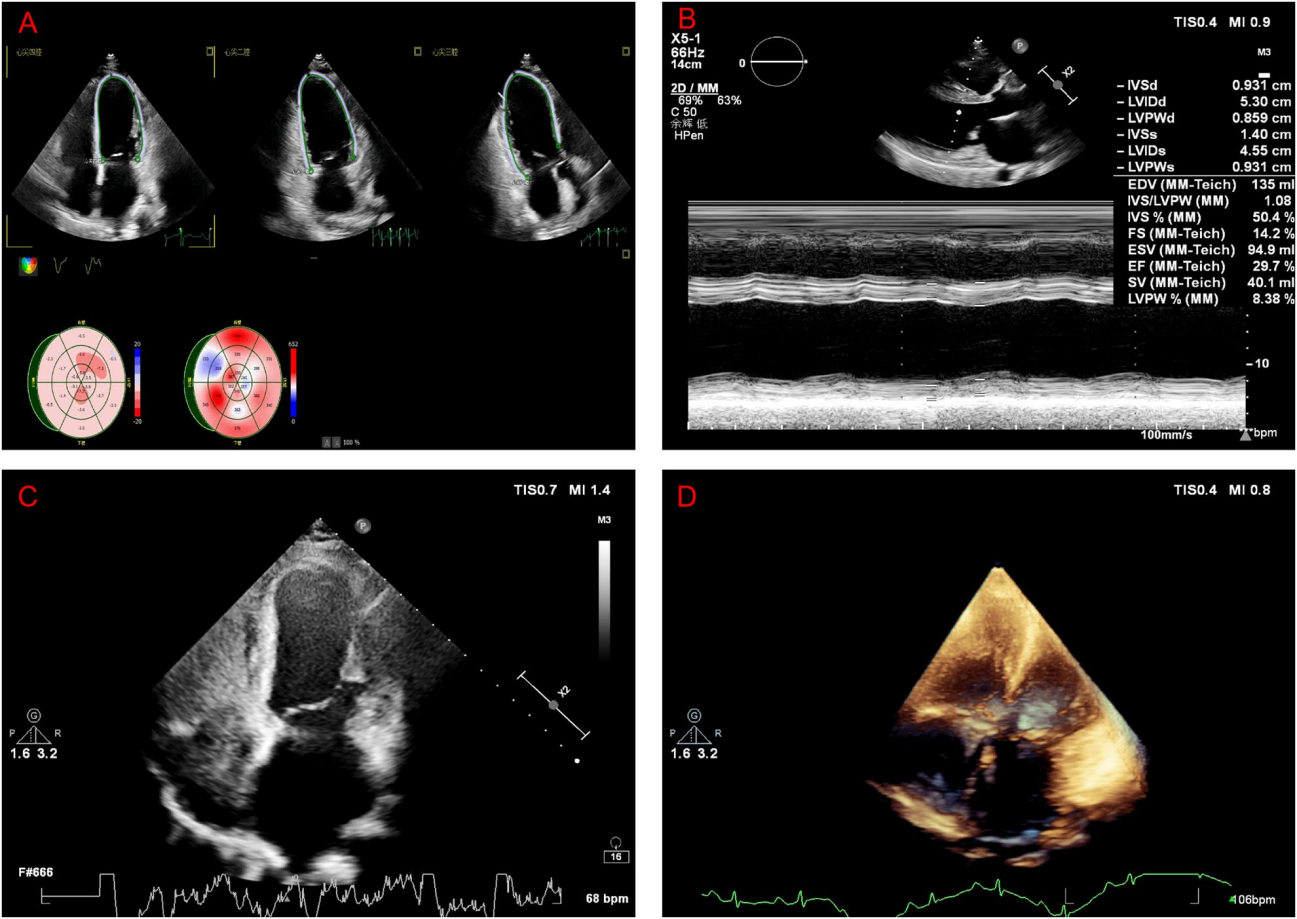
Echocardiography and contrast findings. (A,B) Transthoracic echocardiography showing a dilated left ventricle with diffuse hypokinesia (LVIDd 63 mm, LVEF 30%) in orthogonal views. (C,D) Right heart contrast echocardiography demonstrating no right-to-left shunt at rest or during the Valsalva maneuver.
